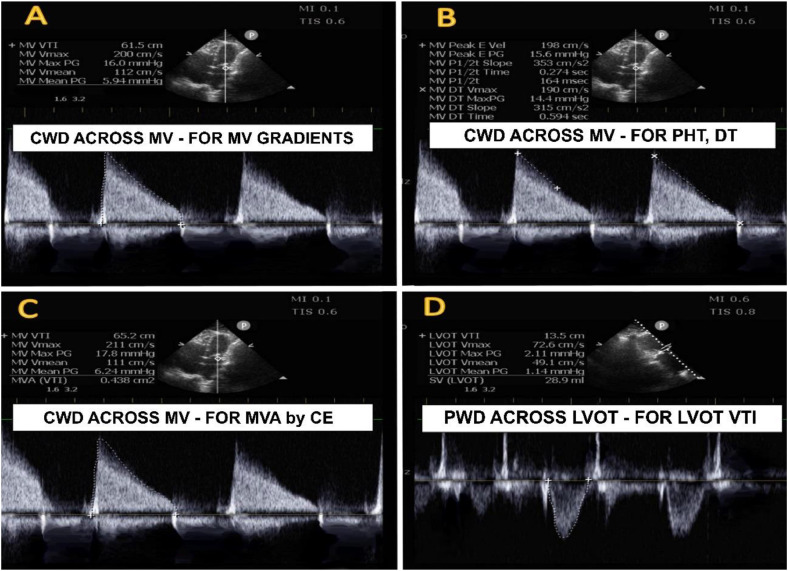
Images showing various echocardiographic parameters to determine the severity of MS. (A) CWD across mitral valve in transthoracic apical four-chamber view-spectral Doppler traced to determine the Vmax-maximum velocity, peak, and mean pressure gradients. (B) CWD across mitral valve in transthoracic apical four-chamber view-spectral Doppler traced along the slope starting from the peak to half the velocity for determination of PHT and till the baseline to derive DT. (C) CWD across mitral valve in transthoracic apical four-chamber view-spectral Doppler traced to obtain MV VTI for the derivation of MVA by continuity equation. (D) LVOT VTI was obtained by tracing the PWD spectral trace across LVOT in an apical five-chamber view. CE, Continuity Equation; CWD, Continuous Wave Doppler; DT, Deceleration Time; LVOT, Left Ventricular Outflow Tract; MV, Mitral Valve; MVA, Mitral Valve Area; PHT, Pressure Half Time; PLAX, Parasternal Long Axis view; PWD, Pulse Wave Doppler; VTI, Velocity Time Integral.
